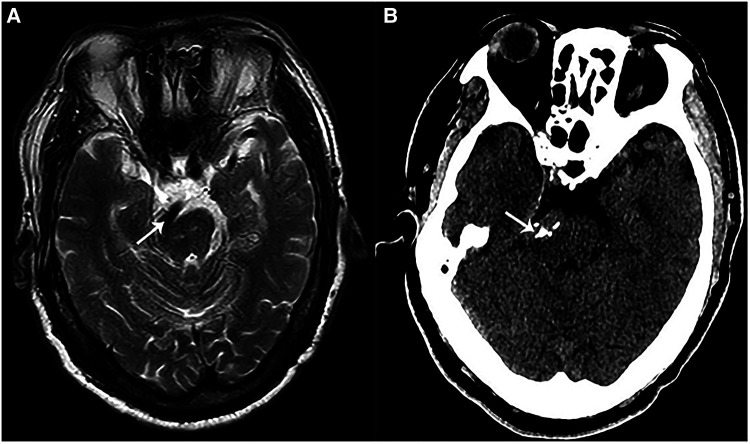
Preoperative imaging of a patient with VBA-associated HFS. (A) A preoperative magnetic resonance imaging (MRI) showed that VBA (white arrow) deformed and compressed the brainstem at pons. (B) A preoperative CT showed calcified VBA (white arrow) which compressed the brainstem at pons.
